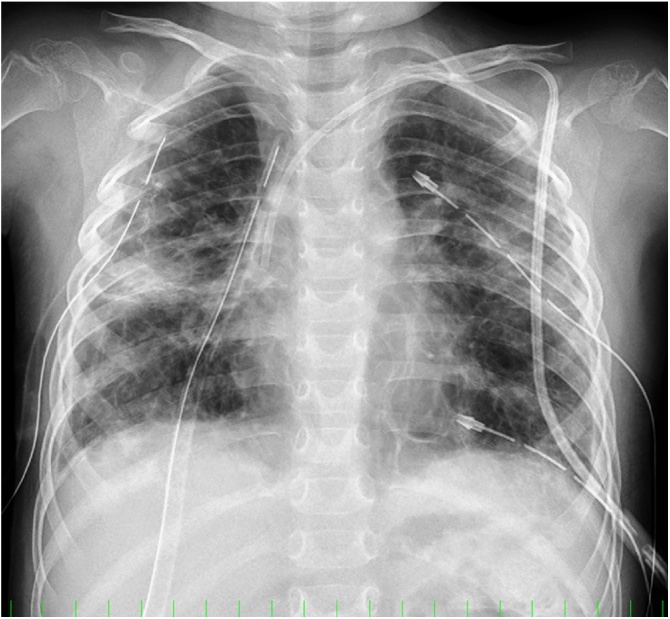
Chest X-ray showing multinumber chest tubes due to repeated bilateral PTX.
